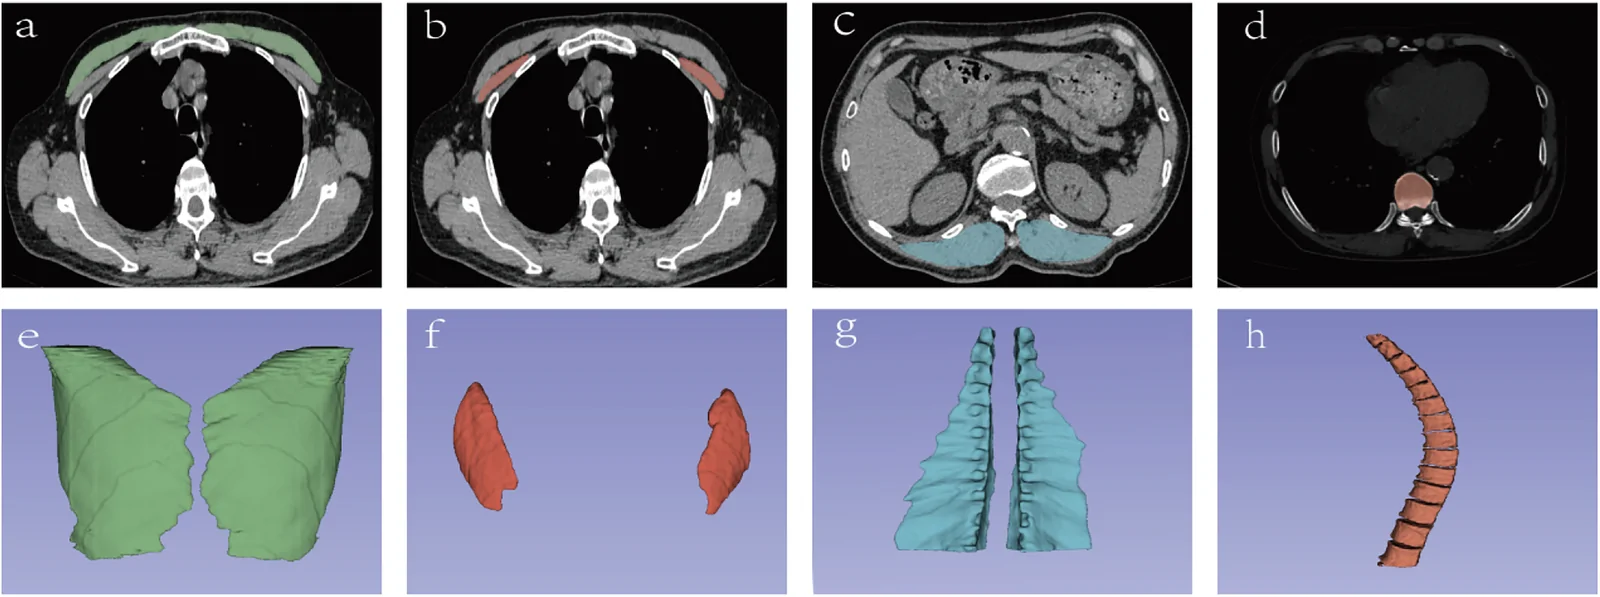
Representative CT images for the measurement of cross-sectional area (CSA) and volume of the pectoralis major (PM_major_), pectoralis minor (PM_minor_), erector spinae (ESM) muscles and vertebral. a, b The CT axial slice of the PM_CSA_ (bilateral PM_major_ are shaded in green and PM_minor_ are shaded in red). c The CT axial slice of the ESM_CSA_ (bilateral ESM are shaded in blue). d The vertebral body CSA (orange-shaded area). e–h PM_major_, PM_minor_, ESM, and vertebral body volume (within the field of view of the chest CT scan)
